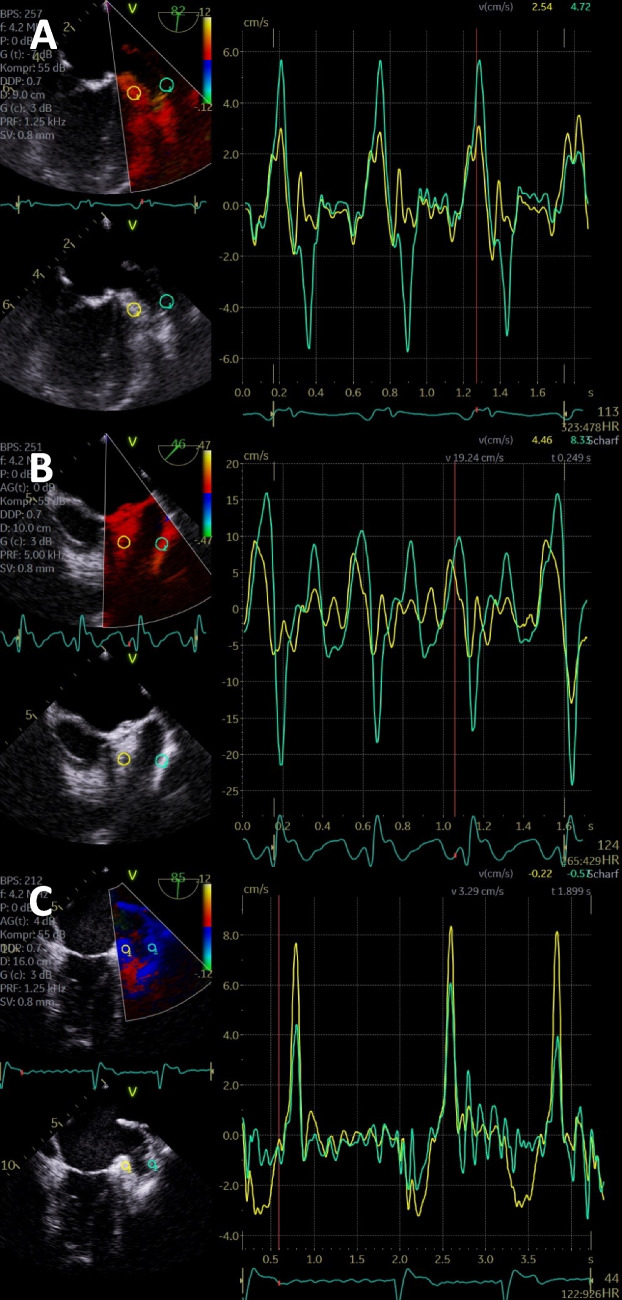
Echocardiographic analysis of the left atrial appendage (LAA) function in patients with atrial fibrillation (AF) or at risk of AF—comparison of simultaneous medial and lateral LAA pulsed wave (pw) tissue Doppler velocities by postprocessing. A Patient with sinus tachycardia. Medial LAA wall velocity is displayed by the yellow curve (region of interest). Lateral LAA wall velocity is displayed by the green curve (region of interest); lateral amplitudes are higher than medial reflecting normal LAA function. B Patient with atrial flutter (124 bpm). Medial LAA wall velocity is displayed by the yellow curve (region of interest). Lateral LAA wall velocity is displayed by the green curve (region of interest). Lateral amplitudes are higher than medial reflecting normal LAA function. C Patient with AF (44 bpm). Medial LAA wall velocity is displayed by the yellow curve (region of interest). Lateral LAA wall velocity is displayed by the green curve (region of interest). Lateral amplitudes are lower than medial reflecting pathological LAA function
